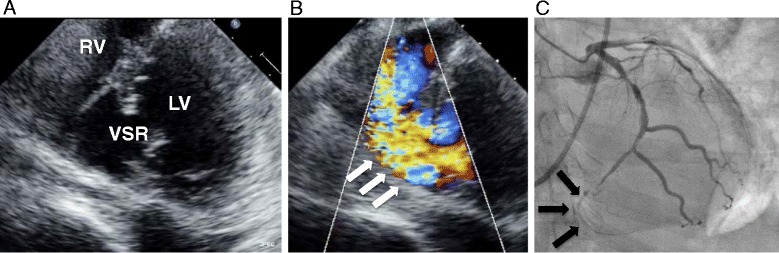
Preoperative examinations. a Short-axis view of preoperative transthoracic echocardiography showing a perforation site (17 mm in diameter) near the posteromedial papillary muscle in the basal ventricular septum. b Left-to-right shunt flow via the ventricular septal rupture in the systolic phase (*white arrow*). c. Coronary angiography showing complete occlusion of the last branch of the dominant left circumflex artery (*black arrow*)
